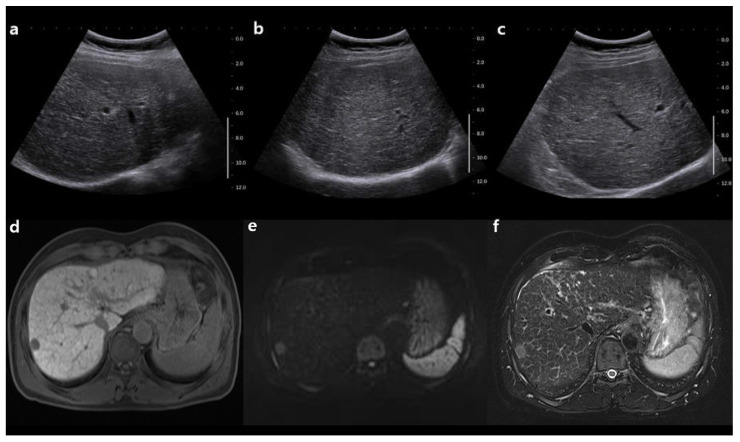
A 64-year-old man with chronic hepatitis B. (a–c) Surveillance ultrasonography does not show any focal hepatic lesion, whereas (d–f) surveillance AMRI shows a 1.5 cm nodule in segment VII. Ultrasonography shows coarse echotexture of the liver (a), but it shows no focal hepatic lesion on the right lobe upper view (b) and lower view (c). The nodule has hypointensity on the hepatobiliary phase image (d), restriction on the diffusion-weighted image (e), and moderate hyperintensity on the T2-weighted image (f). The surveillance AMRI enabled us to make a diagnosis of HCC.
